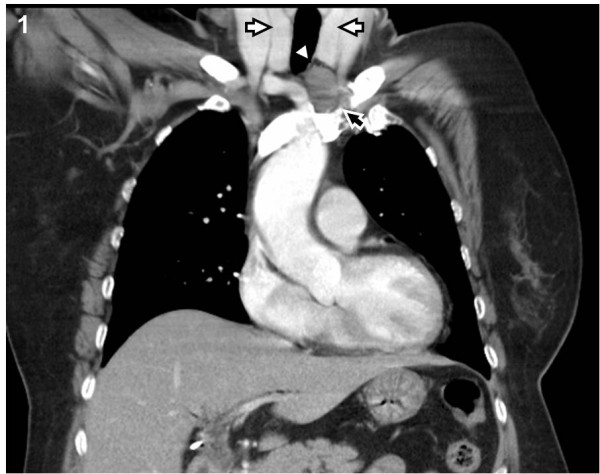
Contrast-enhanced computed tomography of the chest showed a tumor mass (black arrow) at the lower pole of the left thyroid gland (white arrow). The fat plane (arrowhead) between the thyroid gland and the tumor was clear.
